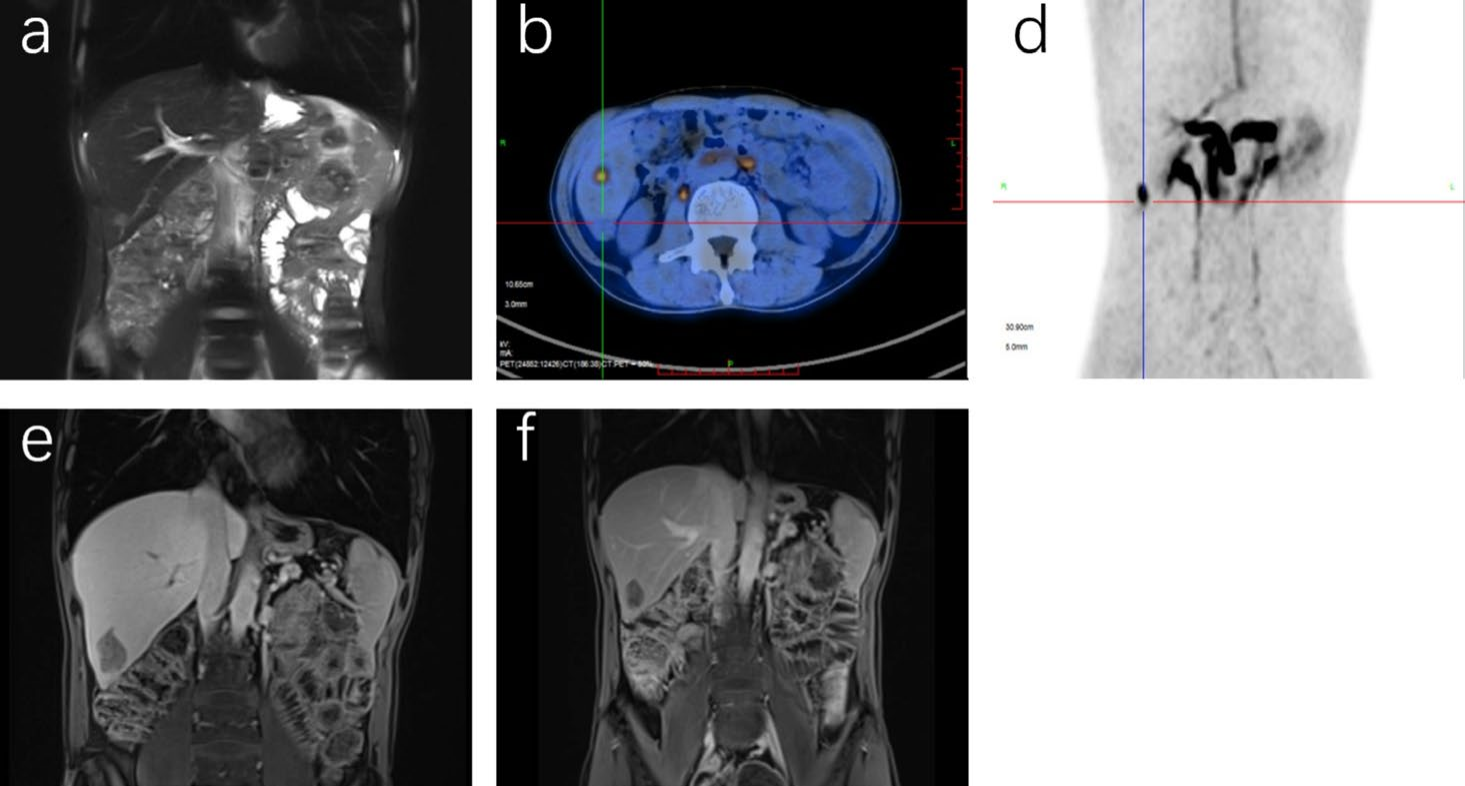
Tumor liver metastasis in 2024. MRI in April 2024 showed a nodule in the inferior segment of the right posterior hepatic lobe, which may represent an additional liver metastasis (a). PET/CT in April 2024 showed a nodule in the right posterior lobe of the liver with increased metabolism, suggesting a new metastasis (b, c). MRI in July 2024 showed that the boundary of the lower segment of the right posterior lobe of the liver was enlarged, which was considered to be a change after the ablation of liver metastases (d). MRI reexamination in September 2024 showed that the nodules in the inferior segment of the right posterior lobe had slightly decreased in size compared to those observed in July 2024 (e, f).
